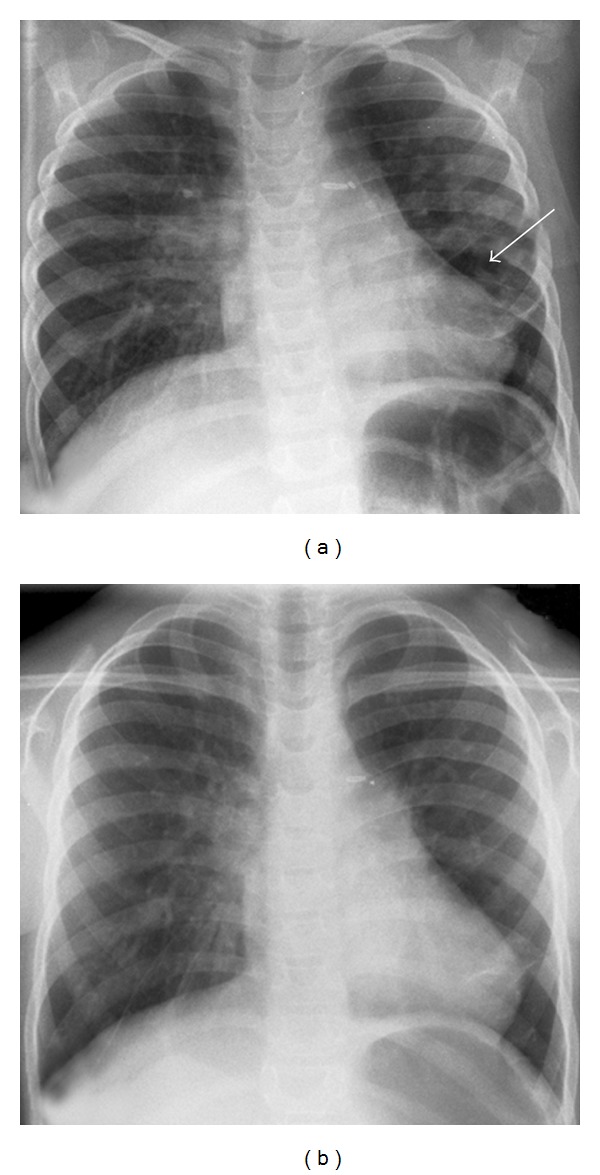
Chest X-ray of patient 2 in anterior-posterior condition, (a) Chest X-ray at the age of 3 years: there are signs of hyperlucent and hyperinflation with cystic-imaging areas (→) and laterally ascending ribs as a sign of lung inflation. In some areas of the lungs, there are consolidations. The heart silhouette is enlarged and clips are present in typical location after surgical closure of a patent ductus arteriosus, (b) Chest X-ray at the age of 5.5 years: persistent signs of bronchial obstruction. Chronic consolidations are found perihilar at the right side and behind the heart.
